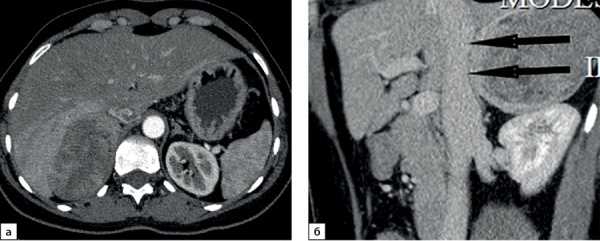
Рисунок 14. Компьютерные томограммы пациентки М., 41 года, с крупной опухолью правого надпочечника размерами до 90 мм: а — артериальная фаза постконтрастного сканирования, диффузно неравномерное накопление контрастного препарата, зона гиперперфузии в компримированном отделе правой доли печени; б — изображение, реформатированное в косой сагиттальной плоскости, венозная фаза сканирования; образование широко прилежит, деформирует нижнюю полую вену (стрелки). Во время операции потребовалось выполнение пластики нижней полой вены вследствие инвазии в последнюю опухоли надпочечника.Figure 14. Computed tomography of patient M., 41 years old, with a large tumor of the right adrenal gland up to 90 mm in size: a — arterial phase of post-contrast scanning, diffusely uneven accumulation of the contrast agent, hyperperfusion zone in the compressed section of the right lobe of the liver; b — image reformatted in the oblique sagittal plane, venous phase of the scan; the mass is widely adjacent, deforming the inferior vena cava (arrows). During the operation, plastic surgery of the inferior vena cava was required due to invasion into the last tumor of the adrenal gland.
